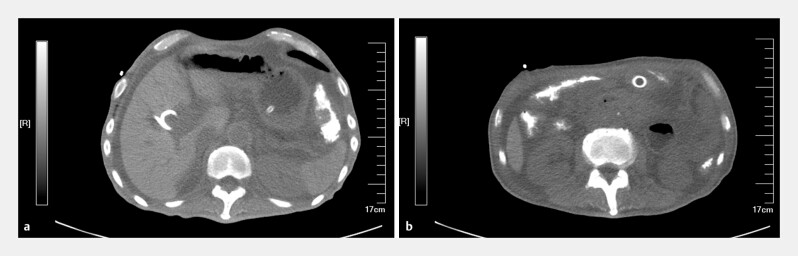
Postoperative abdominal computed tomography.
